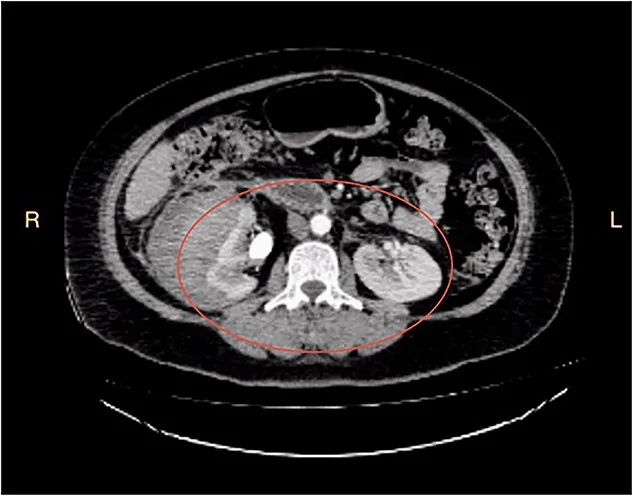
CT urography (axial view). Right kidney demonstrating a homogeneous parenchyma, adjacent to an irregular, heterogeneous mass with predominant hyperdensity compressing the renal parenchyma. A well-defined oval, hyperdense lesion is visible within the renal pelvis (circle). *Source: Image obtained by the authors.* Adjacent to this lesion, a well-defined, irregular, heterogeneous retroperitoneal mass was identified (45 HU) with alternating zones of fluid density (11 HU) and an estimated volume of 577 cc, exerting a significant mass effect on the right kidney and associated with mild perirenal fat stranding.
